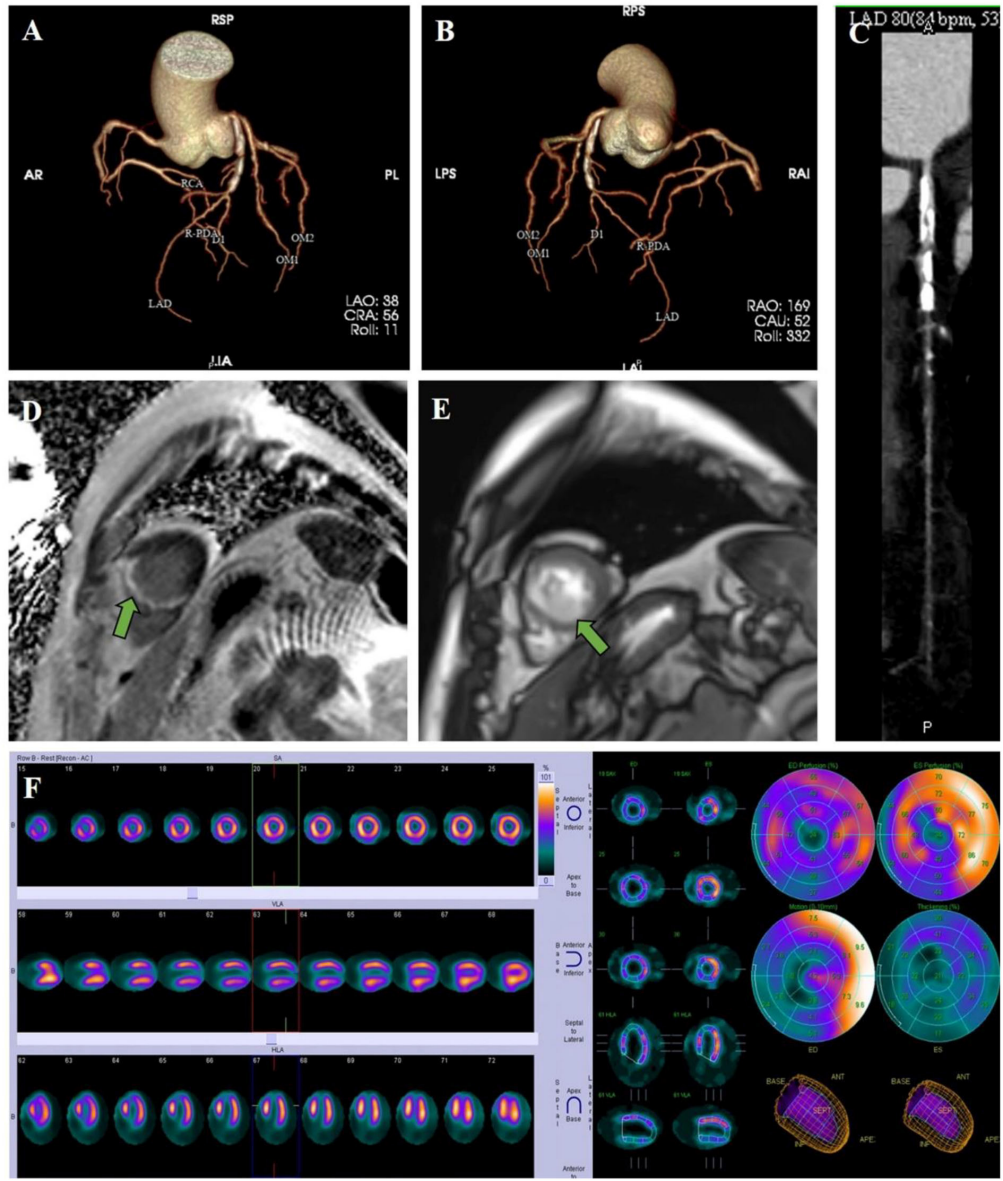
Clinical imaging. (A–C) A coronary CT scan showed that the proximal anterior descending artery had severe calcification and stenosis. (D) Cardiac magnetic resonance imaging revealed delayed enhancement on the apical septal and inferior walls, which presented transmural MI. (E) Cardiac magnetic resonance imaging confirmed the presence of a thrombus at the apex. (F) Resting myocardial radionuclides showed transmural MI and blood perfusion decrement.
